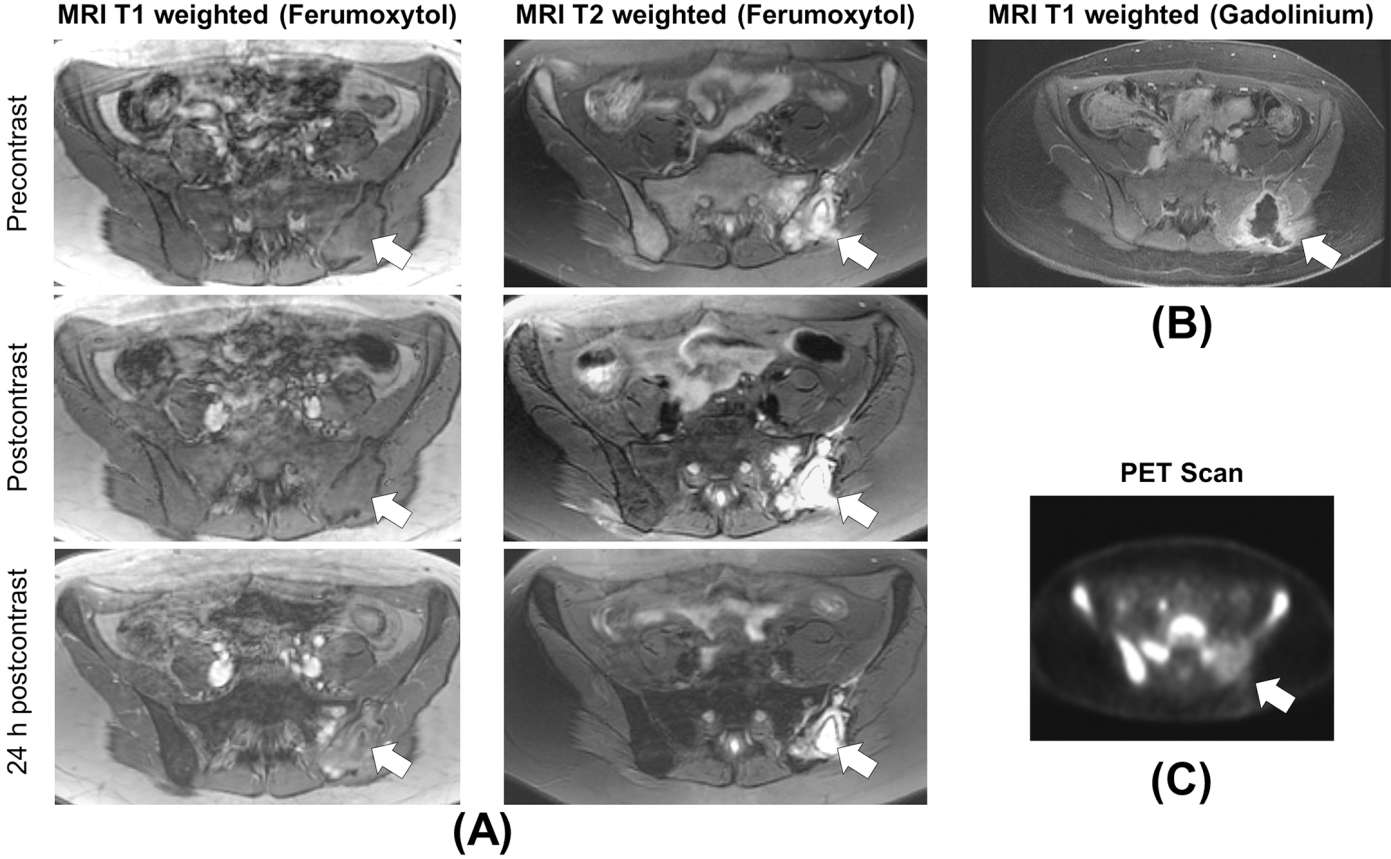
T1-enhancement of ferumoxytol in early necrosis of a Ewing’s sarcoma. A) T1- and T2 weighted MRI images of a 21 year old patient with metastatic Ewing’s sarcoma in the left sacrum and ilium (arrow). Normal bone marrow demonstrates marked T2-enhancement and minimal or no T1-enhancement at 24 h p.i., consistent with intracellular iron in bone marrow macrophages. Fluid-isointense liquefied necrosis demonstrates minimal peripheral rim enhancement. Blood in iliac vessels and T2-hyperintense tumor necrosis in the sacrum demonstrate marked T2-enhancement and marked T1-enhancement at 24 h p.i., consistent with extracellular iron. B) T1 weighed axial MRI image after intravenous injection of Gd-BOPTA demonstrates peripheral rim enhancement around the liquefied necrosis in the ilium, but interestingly no enhancement of the necrotic tumor area in the sacrum. C) PET image from the same area demonstrates highly cellular bone marrow after induction chemotherapy and relatively mild ^18^F-FDG uptake into the tumor (arrow).
